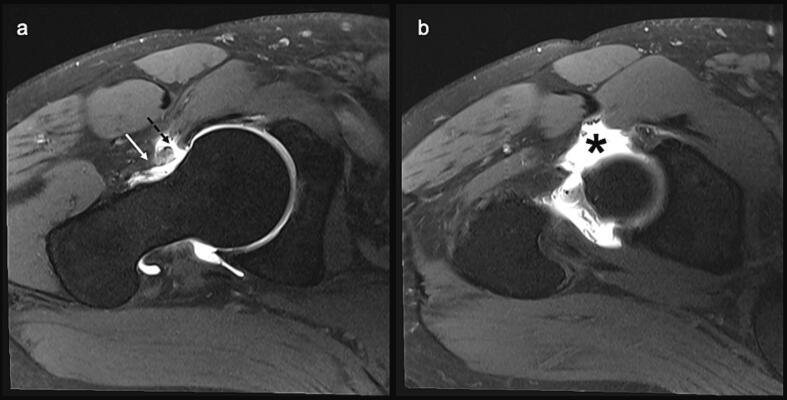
MRI arthrogram of the right hip showing (a) disruption of the joint capsule in the upper anterior region (interrupted arrow) with bulging (solid arrow) and (b) leakage of contrast to the antero-superior aspect of the hip (asterisk).
